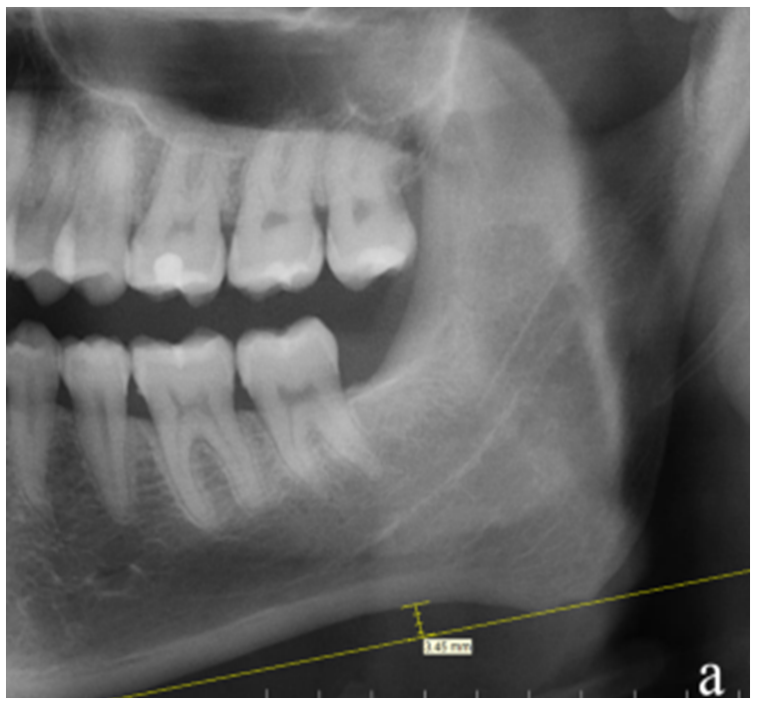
Graphic and radiographic representation for the calculation of the antegonial notch depth, which is calculated by measuring the depth of the notch from the line drawn tangent to the inferior border of the mandible.
